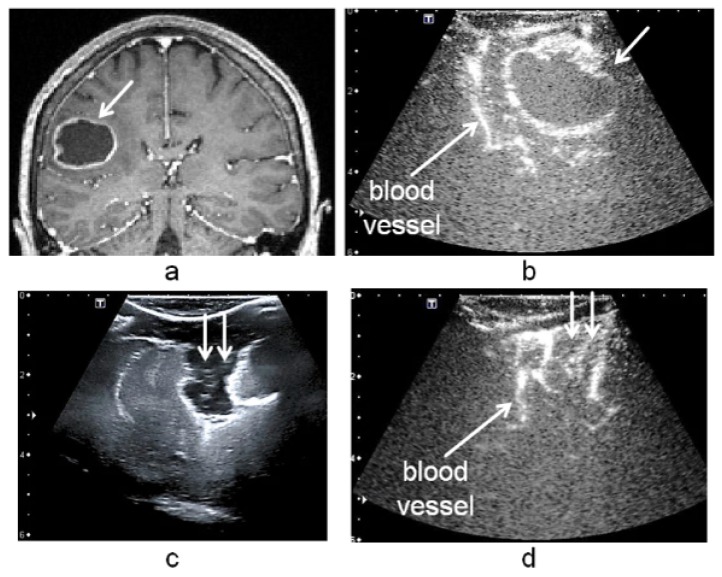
Patient image data acquired during tumor resection. The brain tumor (white arrow) is represented in the preoperative cT1MR data (a) and in the 3D-iCEUS${}_{start}$ (b) acquired at the beginning of the operation. After tumor removing, the resection cavity (indicated by two white arrows in (c) and (d) is well visible in the B-mode ultrasound image (c). In the 3D-iCEUS${}_{end}$ acquired at the end of the operation (d); the borders of the cavity can be easily interpreted as a blood vessel.
